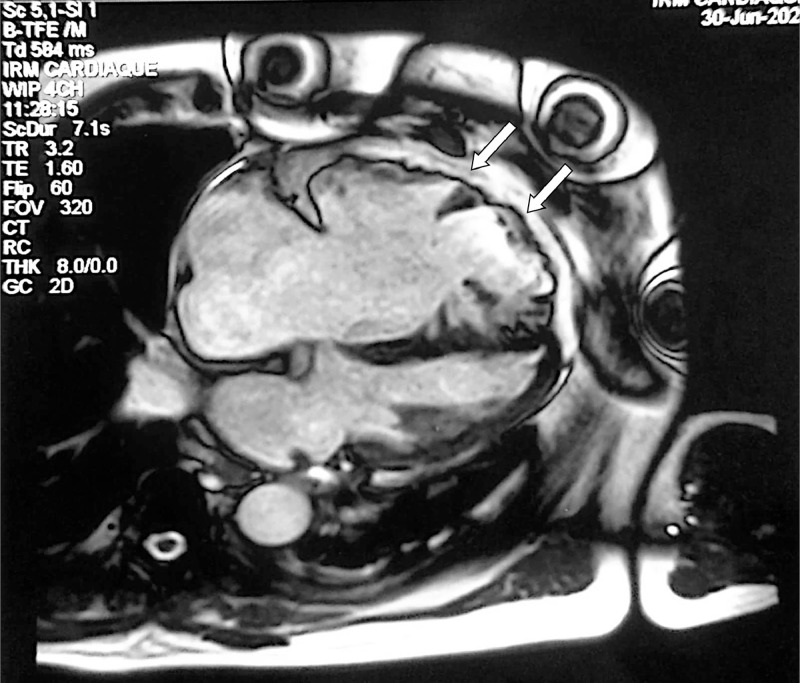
Cardiac MRI cine-MRI sequence incidence 4 cavities showing trabeculations of the apex of the RV (white arrows).
